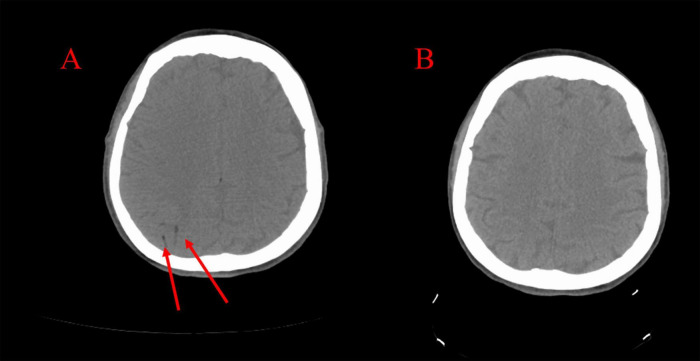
(A) CT scan taken immediately following the stroke demonstrating right-sided cerebral arterial gas embolism. (B) CT scan taken following hyperbaric oxygen therapy demonstrating resolution.
